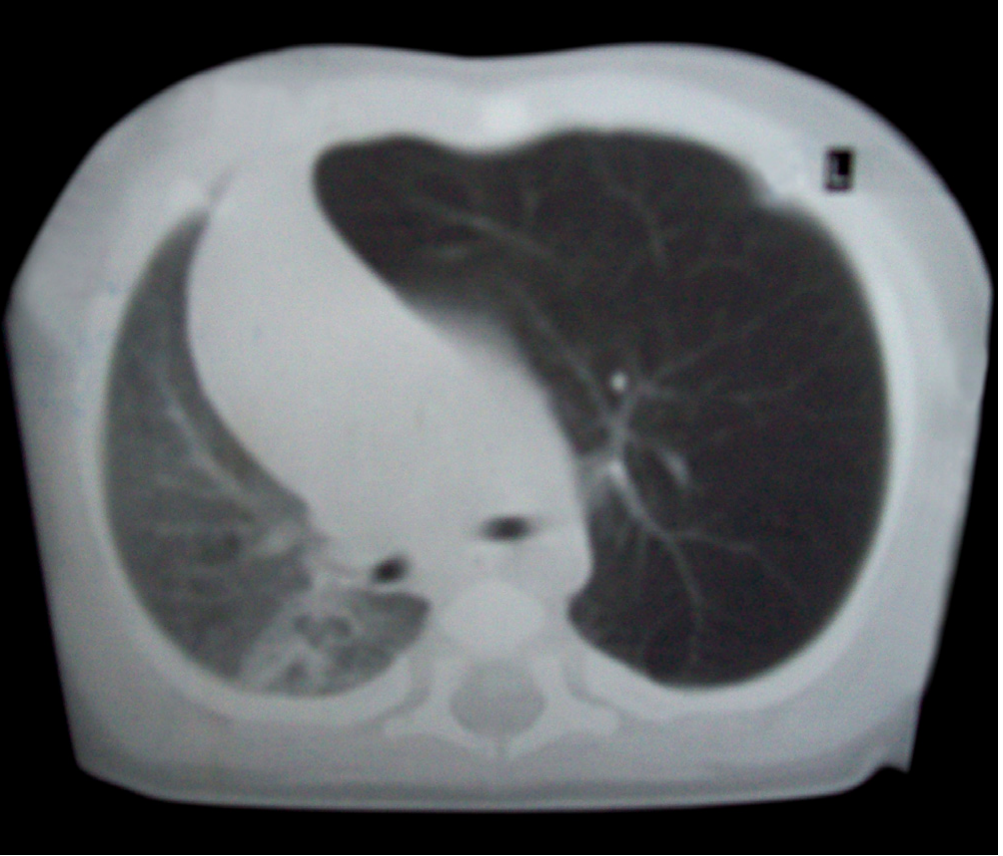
CT scan showed a hyperluscent, hyper extended lobe attenuated but intact pattern of organized vascularity.
